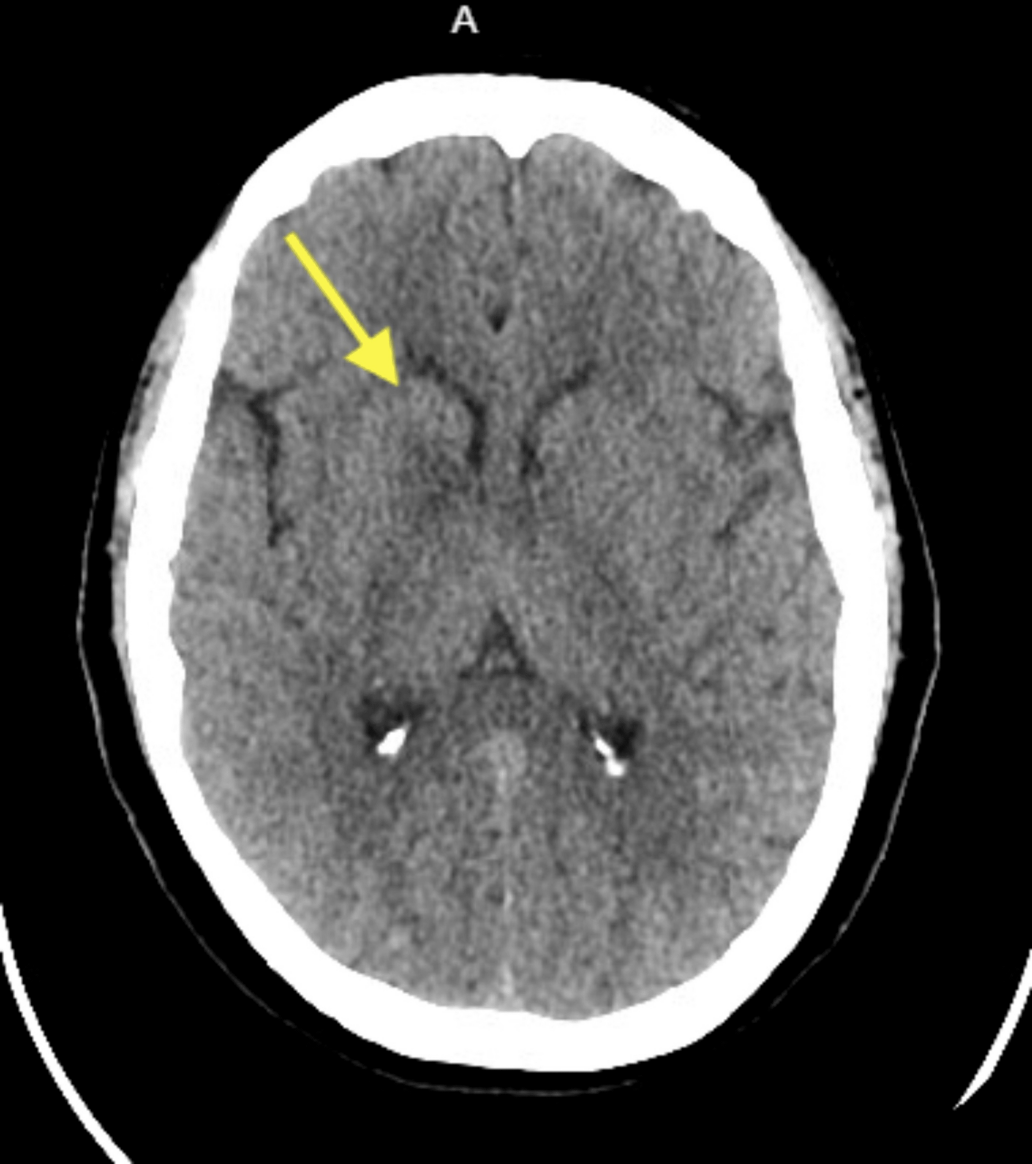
Non-contrast head CT revealing a right basal ganglia lacunar hypodensity suspicious for an acute infarct (yellow arrow).
